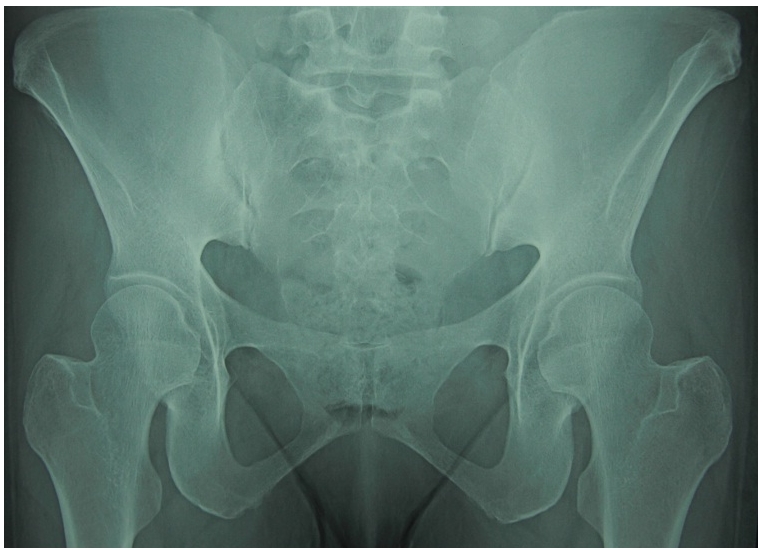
Radiographie du Bassin montant une Sacroilite stade III de Forestier bilatéra;e chez un patient marocain de 45 ans, atteint de Sarcoidose et spondylarthrite ankylosante.
